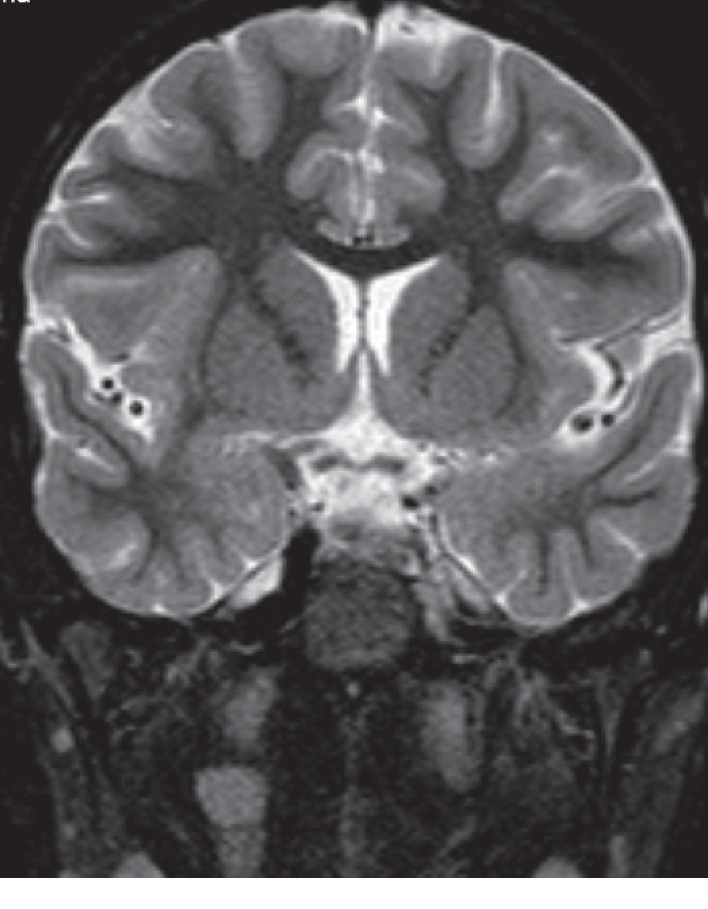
Coronal T2-weighted image shows inflammatory involvement of the left petrous apex and Meckel cavity with petrous carotid stenosis.
